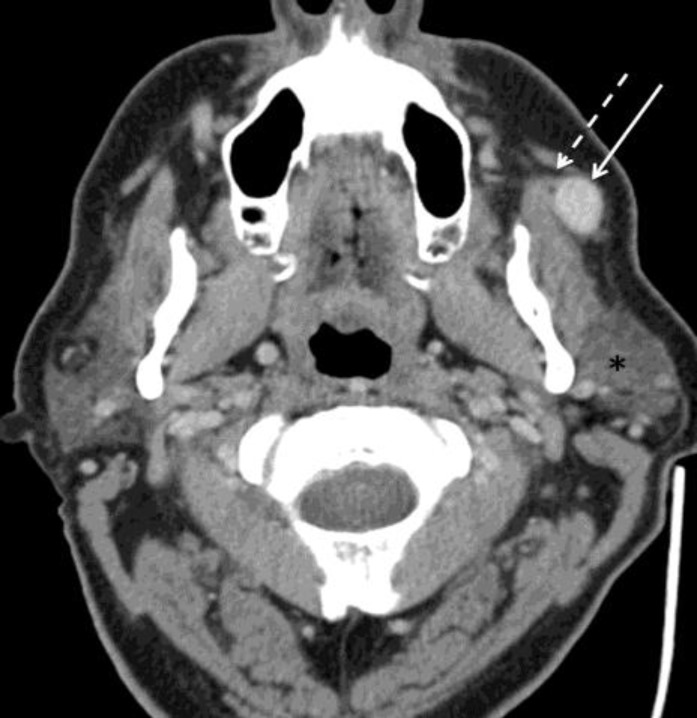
Axial contrast-enhanced CT image demonstrates a solid avidly enhancing nodule (white solid arrow) superficial to the masseter muscle and deep to the platysma. It is intimately associated with the parotid duct (dashed white arrow). The mass is anterior to the parotid gland (*).
